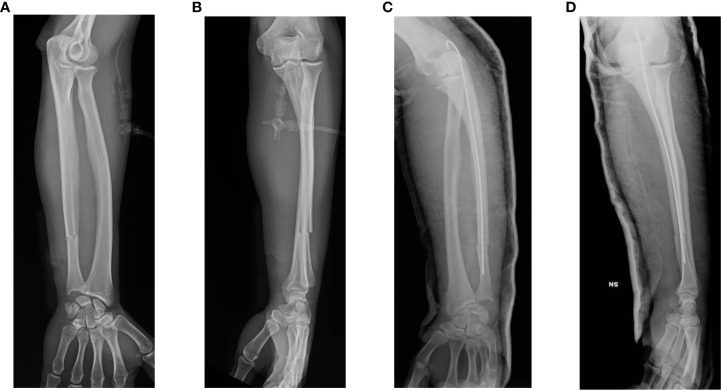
Pre- and post-operative imaging of the patient’s left forearm fracture. Preoperative anterior–posterior (A) and lateral (B) radiographs show the complete fracture of the left distal ulna. Postoperative anterior-posterior (C) and lateral (D) X-rays the day after internal fixation surgery.
